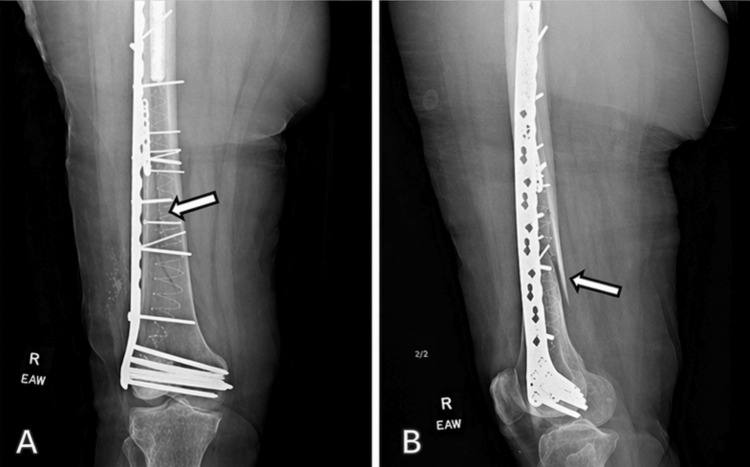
Immediate postoperative radiograph demonstrating IlluminOss intramedullary implantation with subsequent plate and screw fixation The fracture is well reduced with good anatomical alignment.
